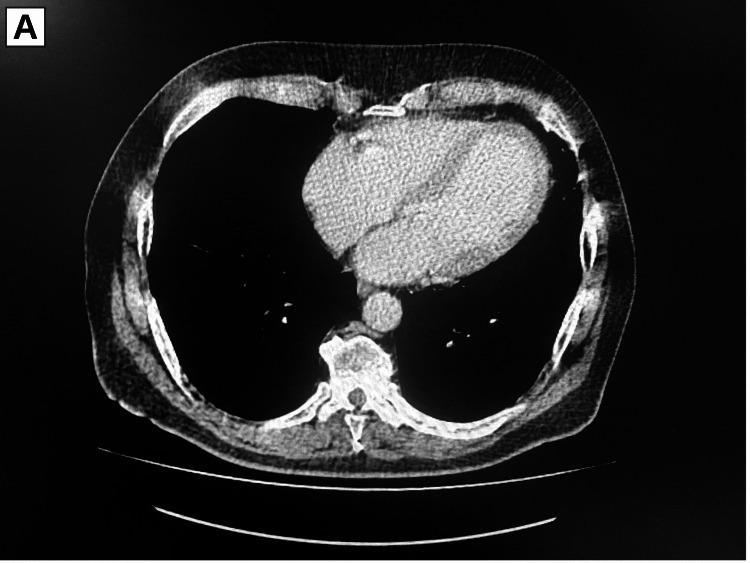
Initial CT Imaging Initial CT of the chest, abdomen, and pelvis. Axial thoracic section on lung windows. The study found no acute intra-abdominal pathology and no mycotic aneurysm; incidental findings were bilateral lower lobe atelectasis, a sub-centimetre left upper lobe pulmonary nodule in keeping with post-infectious change, small bilateral renal cysts, and prostatic calcifications.
